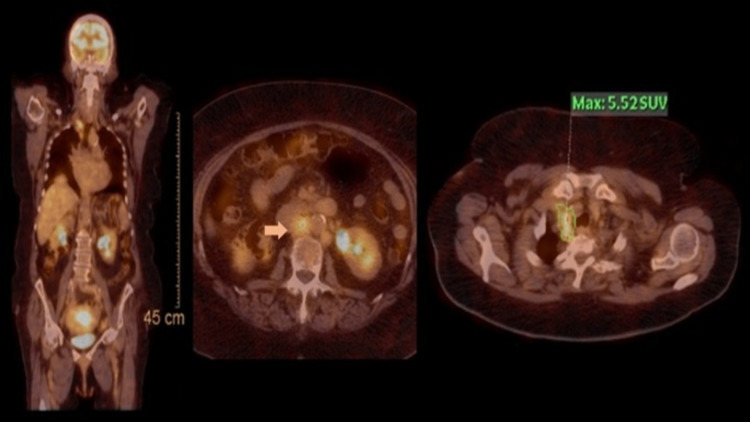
PET/CT from skull to mid-thigh performed in the context of clinical suspicion of EC recurrence Before beginning treatment, an endometrioid carcinoma G2 was diagnosed with the invasion of the outer one-half of the myometrium and vaginal metastasis in a patient. After chemo-radiotherapy, both clinical and radiological responses were obtained. One year post-treatment, the patient performed this PET/CT in the context of recurrence clinical suspicion. A hypermetabolic para-aortic lymph node (arrow) and a right supraclavicular lymph node with a SUVmax of 5.52 (image on extreme right) were detected, conferring the diagnosis of recurrence of the underlying disease.
